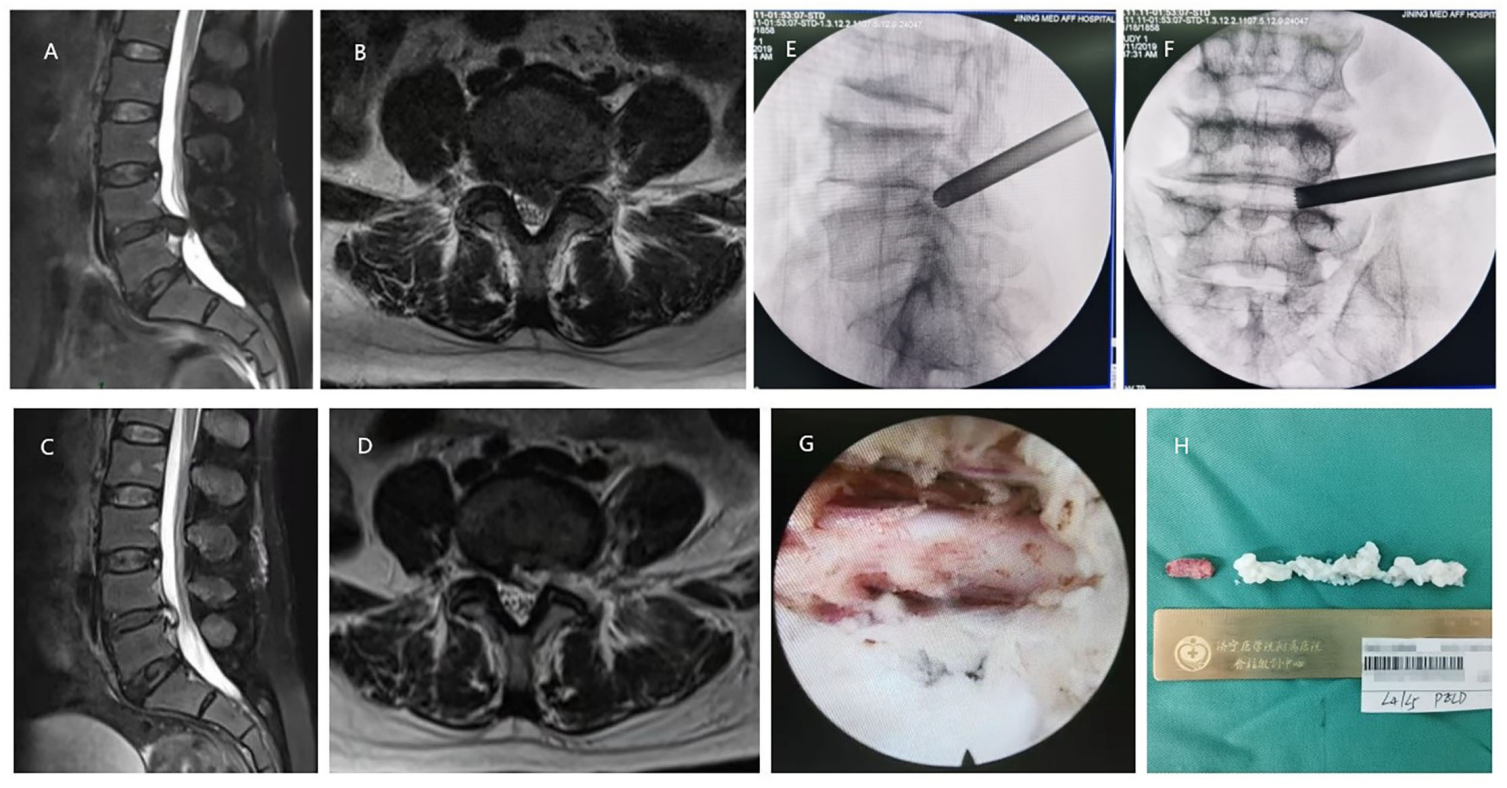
A 67-year-old female with the L4/5 lumbar intervertebral disc herniation (LDH) underwent percutaneous endoscopic lumbar discectomy (PELD) with the approach of transforaminal. (A,B) Preoperative MRI showed LDH was located at the L4/5 level. (C,D) Postoperative MRI revealed the herniated nucleus pulposus tissues were removed and the nerve root was decompressed. (E) Intraoperative C-arm fluoroscopy showed the location of the working channel on lateral film. (F) Intraoperative C-arm fluoroscopy showed the location of the working channel on anteroposterior film. (G) The decompressed nerve root under endoscopic view. (H) Resected nucleus pulposus tissues in the operation.
